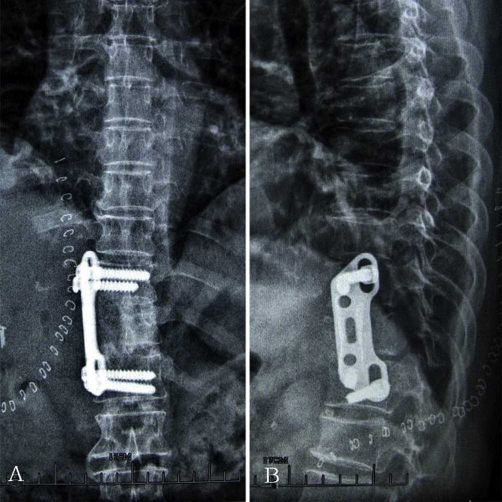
(A) & (B) Anteroposterior and lateral radiograph of thoracic vertebra showed anterolateral decompression and fusion was performed on T10 12.
